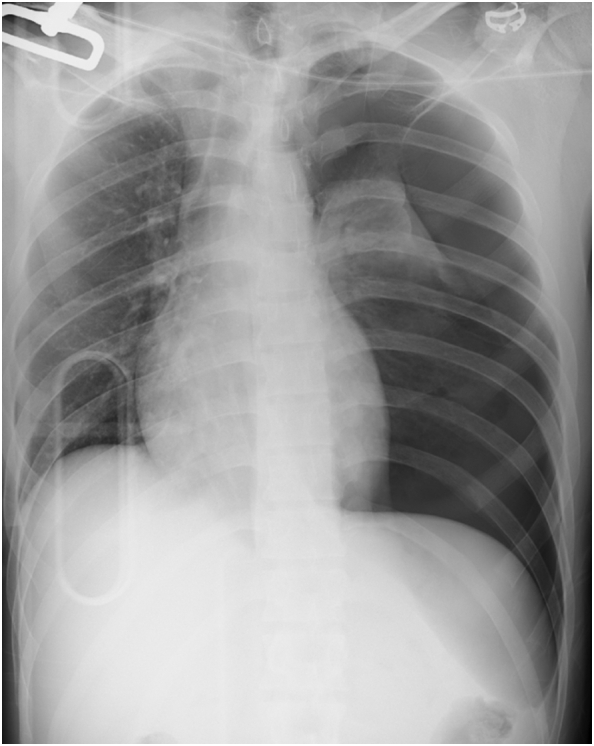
Initial chest X-ray showing left tension pneumothorax. No clear evidence of hemothorax is visible.
